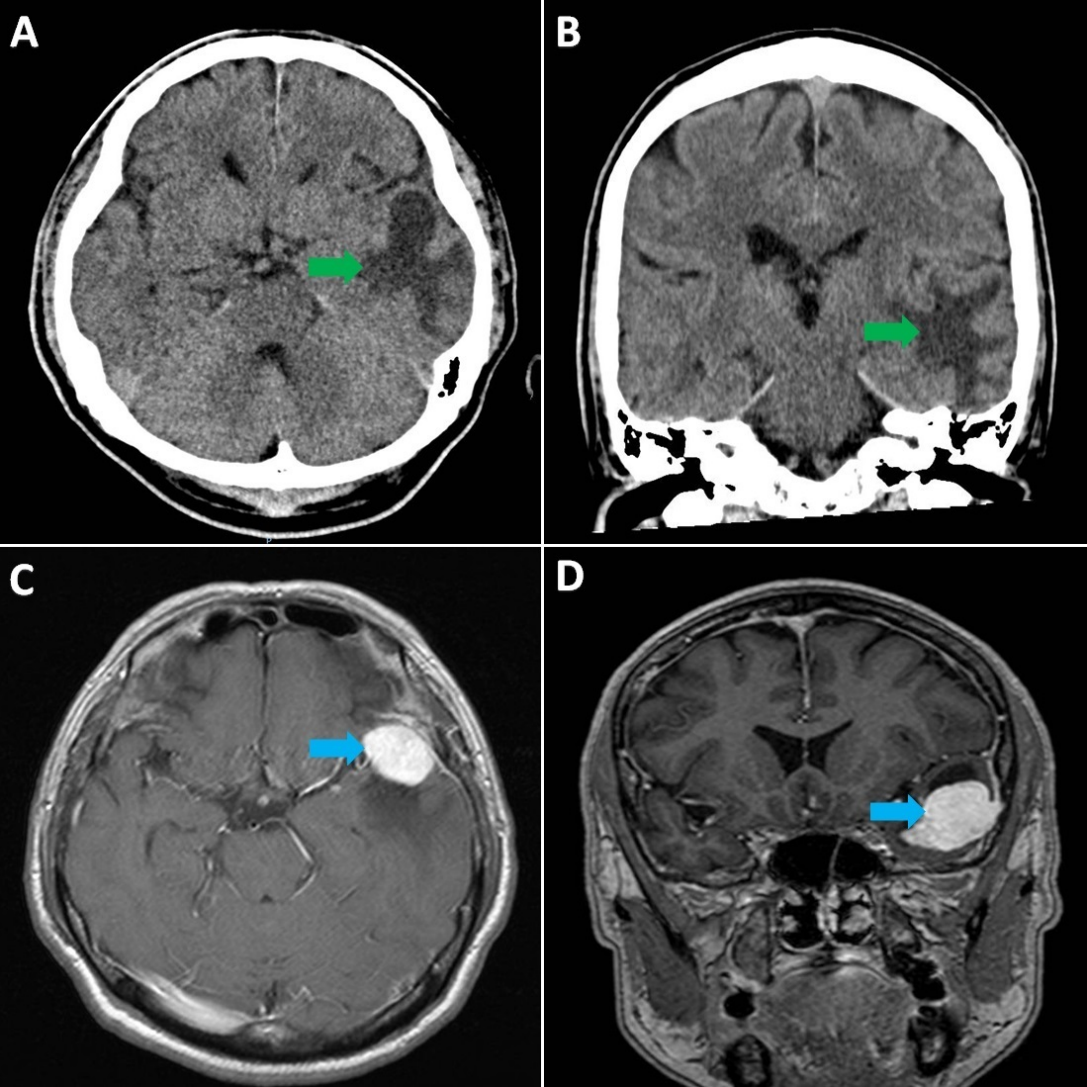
CT scan of head: axial (A) and coronal (B] views, showing suspected mass lesion (green arrows) in the left temporal lobe with surrounding vasogenic oedema and mass effect; MRI of brain: axial [C] and coronal [D] views, showing a well-defined, avidly enhancing extra-axial mass lesion (blue arrows) in the left, middle cranial fossa. CT = computed tomography MRI = magnetic resonance imaging
